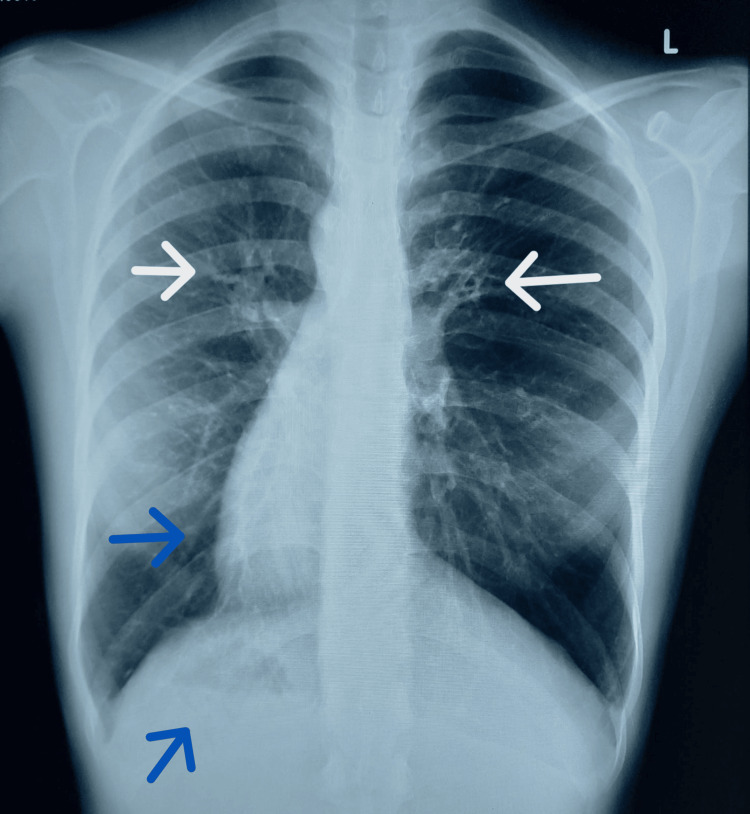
Chest X-ray The chest X-ray showed the cardiac silhouette, aortic knuckle, and gastric air bubble on the right side (blue arrow), suggesting situs inversus, with bilateral localized opacities and ring-like shadows (white arrow).
